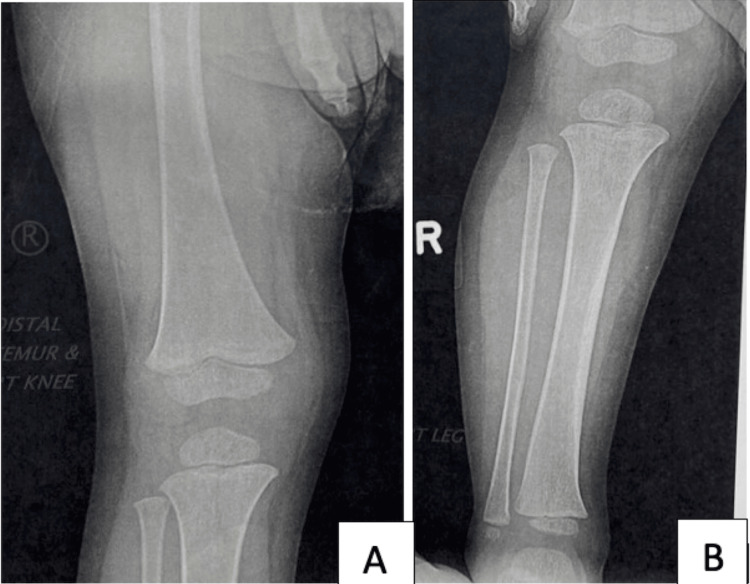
Pediatric patient diagnosed with scurvy. (A, B) Right lower extremity anteroposterior radiograph of a 29-month-old boy was found unremarkable.
